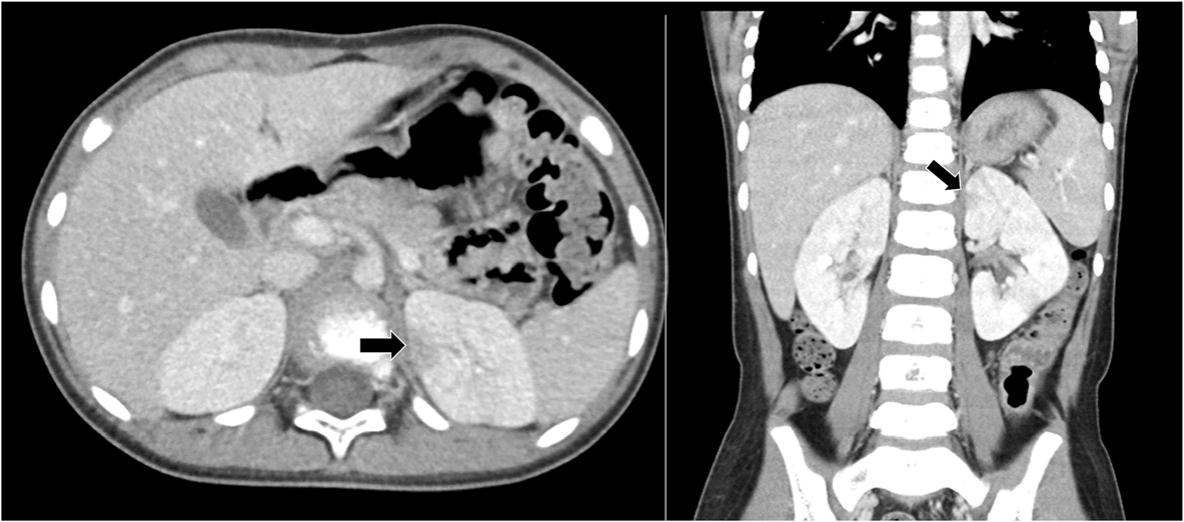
Repeat computed tomography (CT) scan after 3 weeks of meropenem administration. The renal abscess and poor contrast enhancement in the left kidney showed improvement, but poor contrast enhancement remained in in the area where the abscess was located
